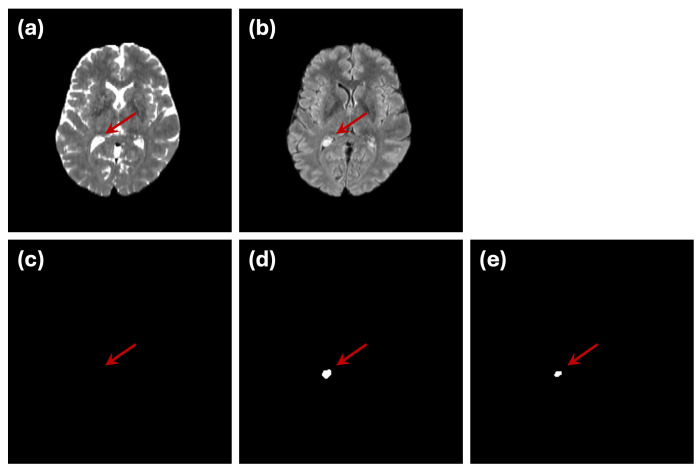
Example of a mismatch between golden truth segmentation and model segmentaion. (a) T2W and (b) FLAIR MRIs indicate a region with high brightness in lateral ventricle (labeled by red arrow) which is not represented in (c) golden truth segmentation but appears in different representations in (d) single (FLAIR) and (e) multi (FLAIR and T2w) modality segmentations. Due to this issue, segmentation accuracy metrics for this subject (UCSF-PDGM-0255) are remarkably low.
